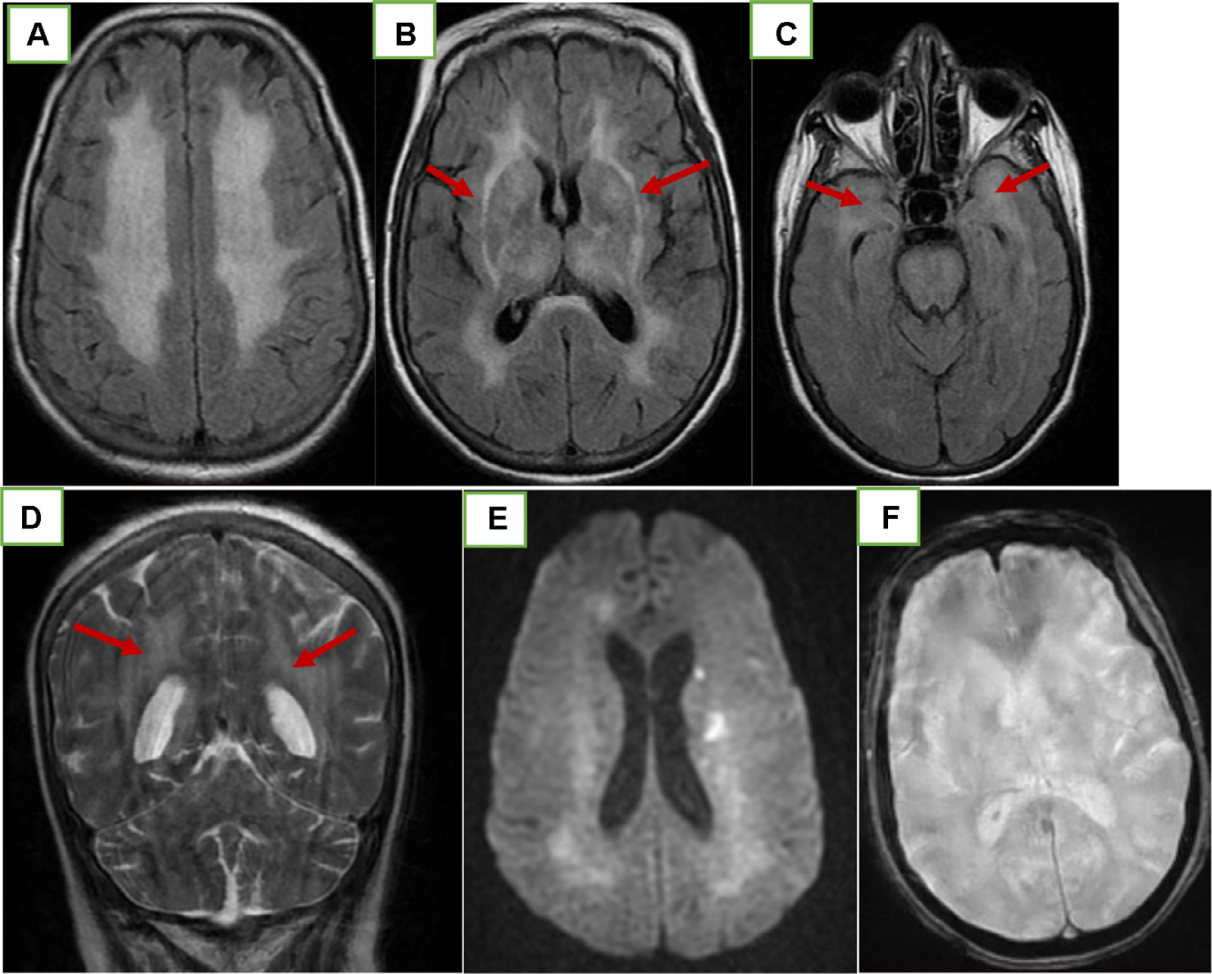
Cranial MRI on axial FLAIR sequence (A to C) and coronal T2 weighted sequence (D) showing increased signal intensity interesting both anterior temporal lobes, the periventricular regions, the subcortical areas, and the external capsules (arrow). DWI image (E) showing a restricted lesion in the left corona-radiata. T2-weighted images (F) did not show microbleeds.
